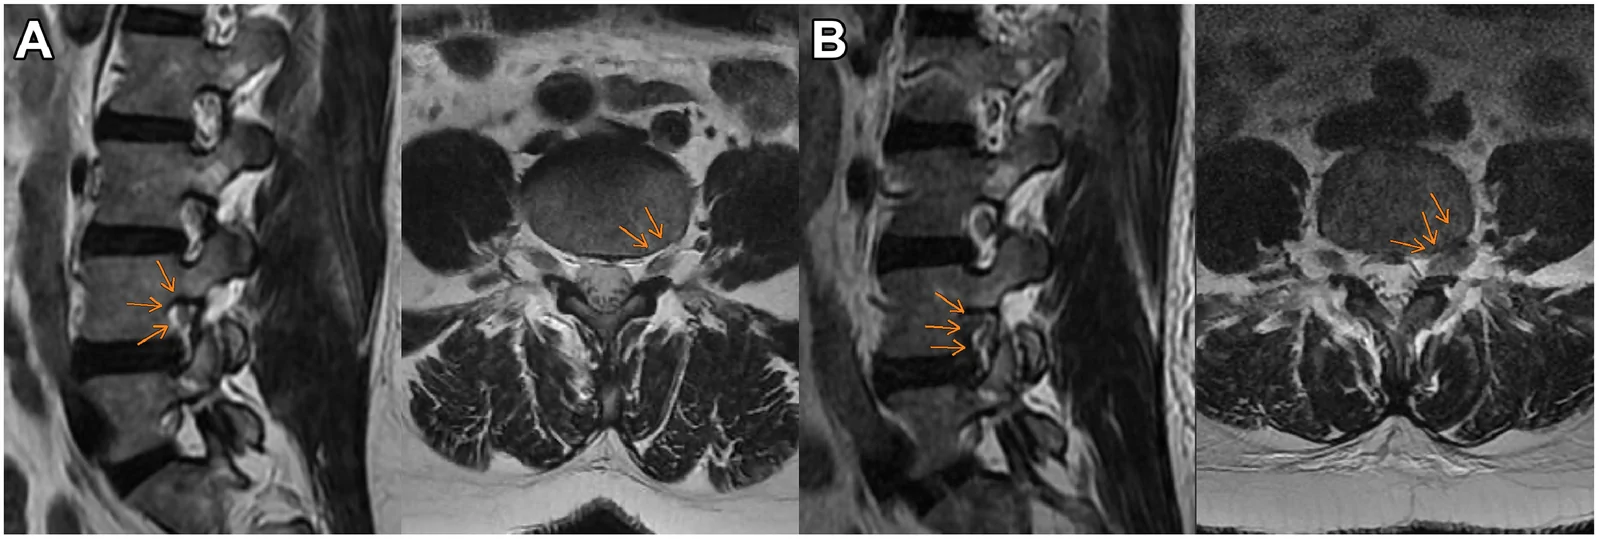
Lumbar magnetic resonance imaging demonstrating progression of L4-L5 disc pathology. (A) Initial study, sagittal and axial T2-weighted images, showing diffuse disc bulging at L4-L5 without significant neural compression (arrows). (B) Repeat study obtained 3 months later, sagittal and axial T2-weighted images at the same level, showing a left foraminal disc extrusion with cranial migration of the fragment into the upper segment of the neuroforamen (arrows), severe foraminal narrowing, and compression with deformity of the exiting left nerve root.
